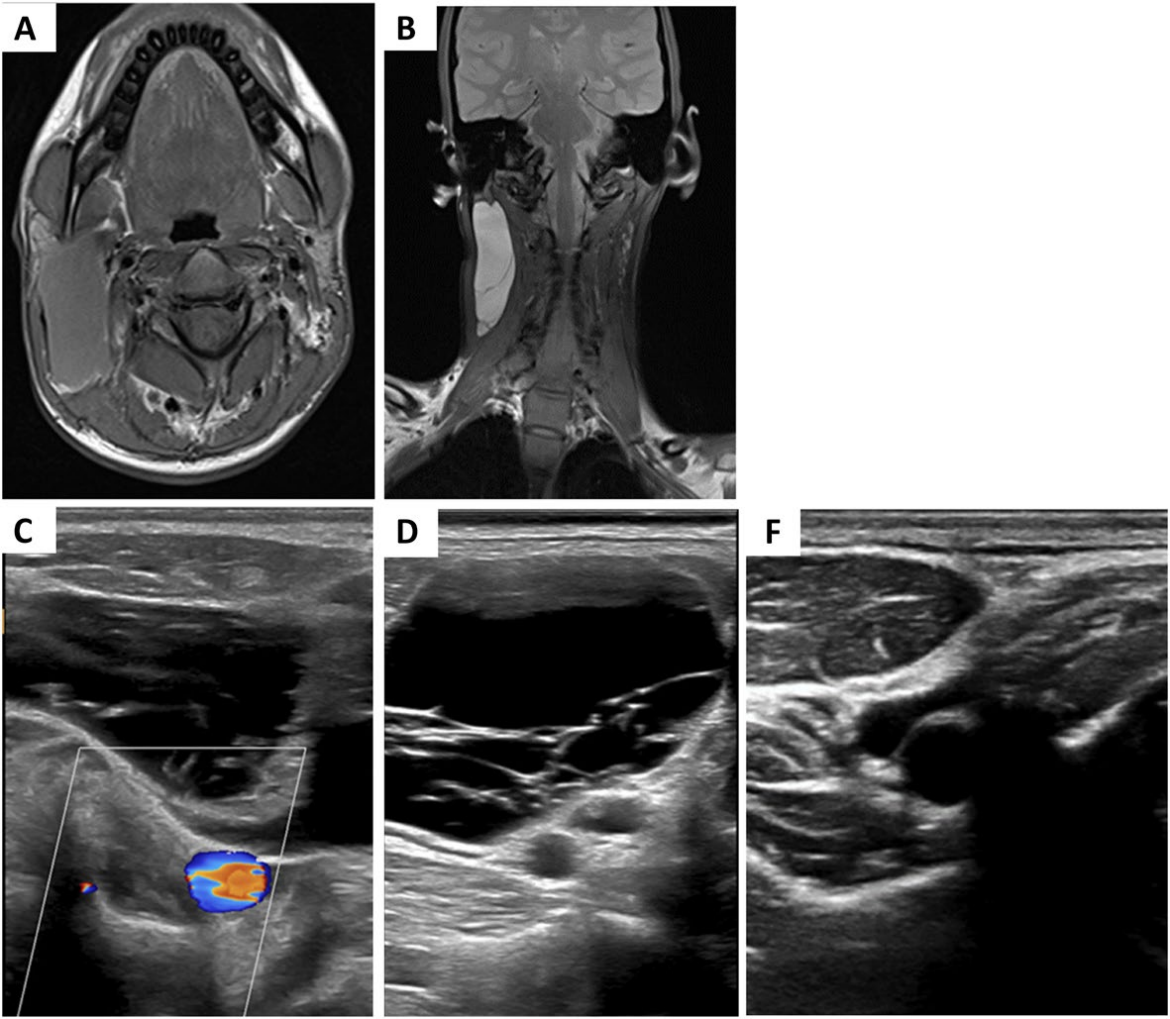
An 18-year-old female patient with a lymphatic malformation was treated with two sessions of sclerotherapy with doxycycline. The patient reported complete resolution of symptoms. A Axial T1WI image reveals well defined hypointense cystic lesion at right side of neck. B Coronal T2WI shows the lesion of high signal intensity. C Doppler US reveals no flow within the lesion in keeping with a low flow vascular malformation with normal flow within the right sided neck vessels. D US image displays multiple internal septations in the lesion. E Complete resolution of the lesion after two sessions of sclerotherapy
